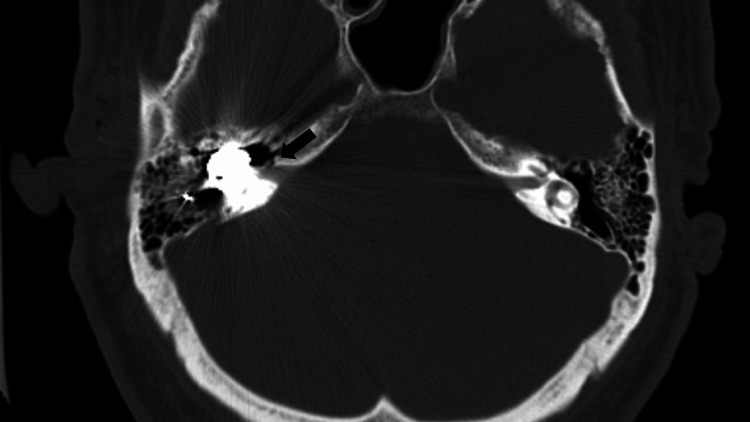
Impacted bullet in ear CT image Axial view of the ear CT scan, showing the foreign body lodged in the middle and inner ear on the right side reaching the most lateral part of the inner auditory canal (black arrow), with destruction of the cochlea and semicircular canals.
